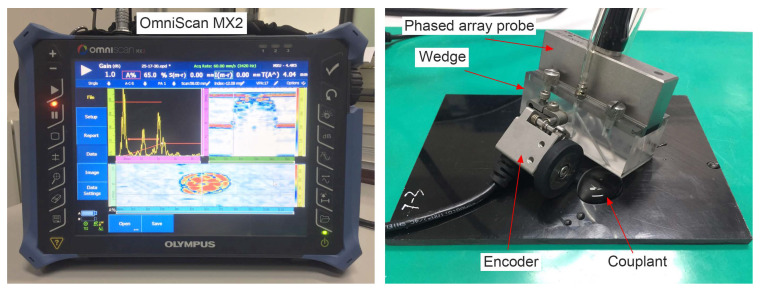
Phased array ultrasonic system.
